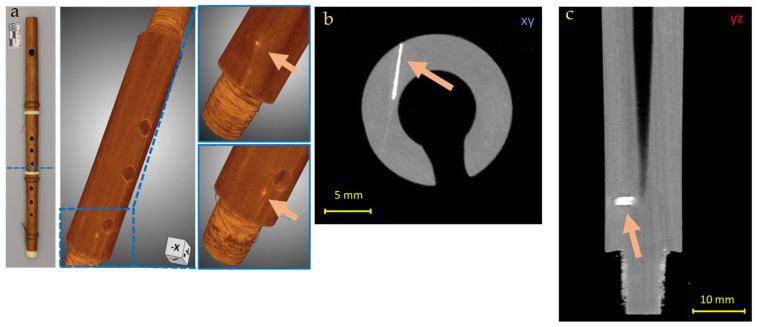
Original and 3D render of the Piccolo Flute body (a) and CT horizontal (b) and lateral (c) slices of the Piccolo Flute body, in which denser filling material is clearly visible inside the wood (orange arrows in (b,c)). In (a) the areas in which the denser material is present (blue line and square) are highlighted.
